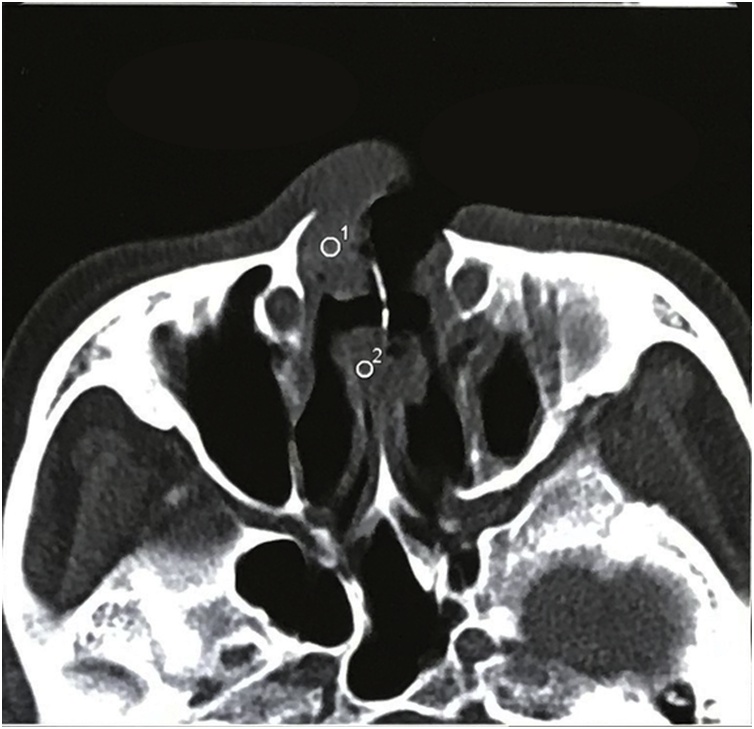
Axial computed tomographic scan showing destruction of the nasal septum, thickness of the maxillary sinus.
